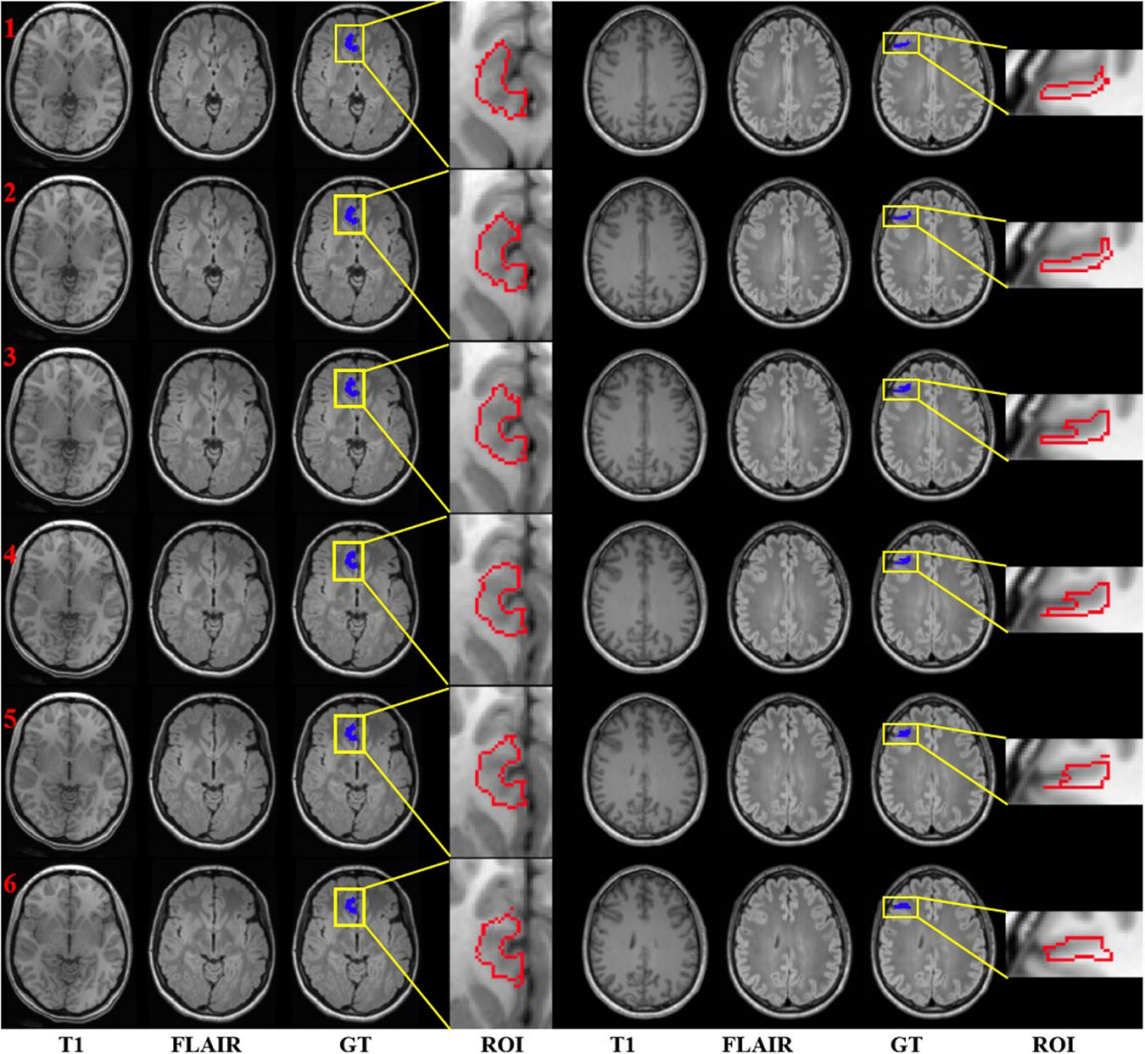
FCD lesion visualization on 6 consecutive MR slices of an MRI-positive patient in columns 1–4 and an MRI-negative one in columns 5–8. The lesion mask is rendered in blue. Region-of-interest (ROI) inside the yellow bounding box surrounding the lesion is enlarged on the right side, with the lesion contour drawn in red
